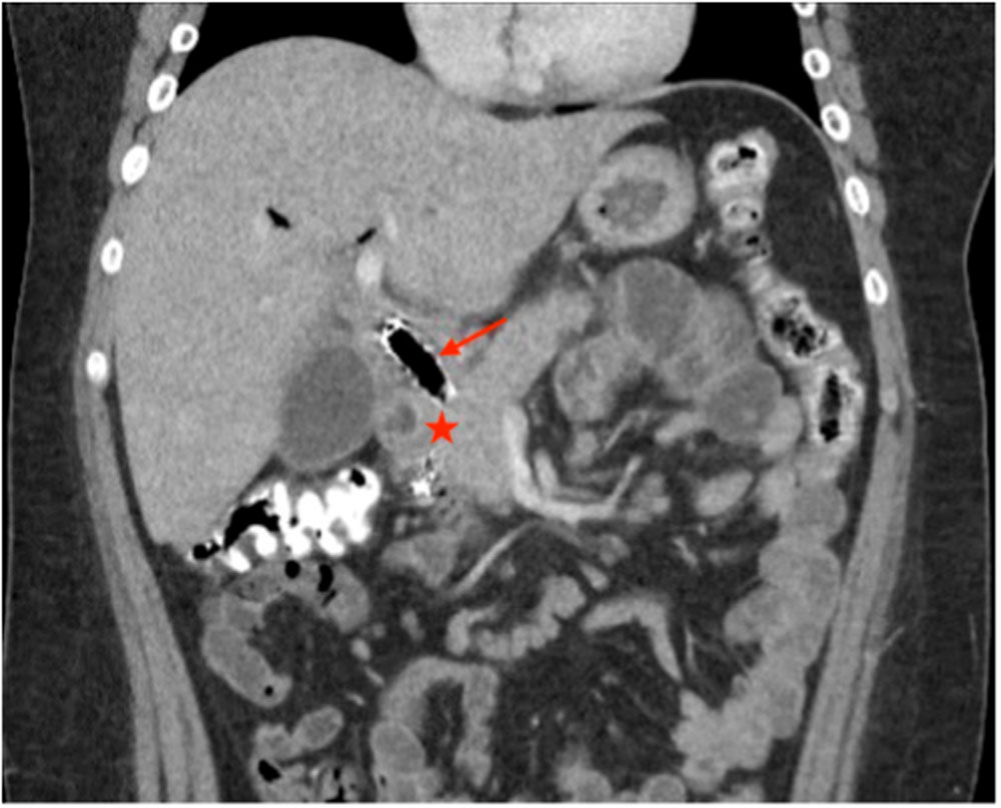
Computed tomography abdomen with contrast. Hypo‐enhanced lesion in head of pancreas (star) with mass effect on the bile duct stent (arrow). Pancreatic head mass measures up to 14 mm.
